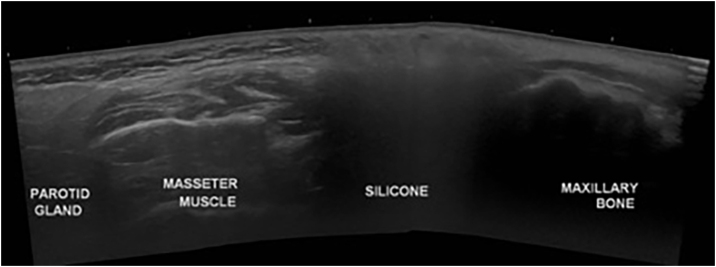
Transverse panoramic ultrasound image of the right cheek with the characteristic “snowstorm” sign between the masseter muscle and maxillary bone.
